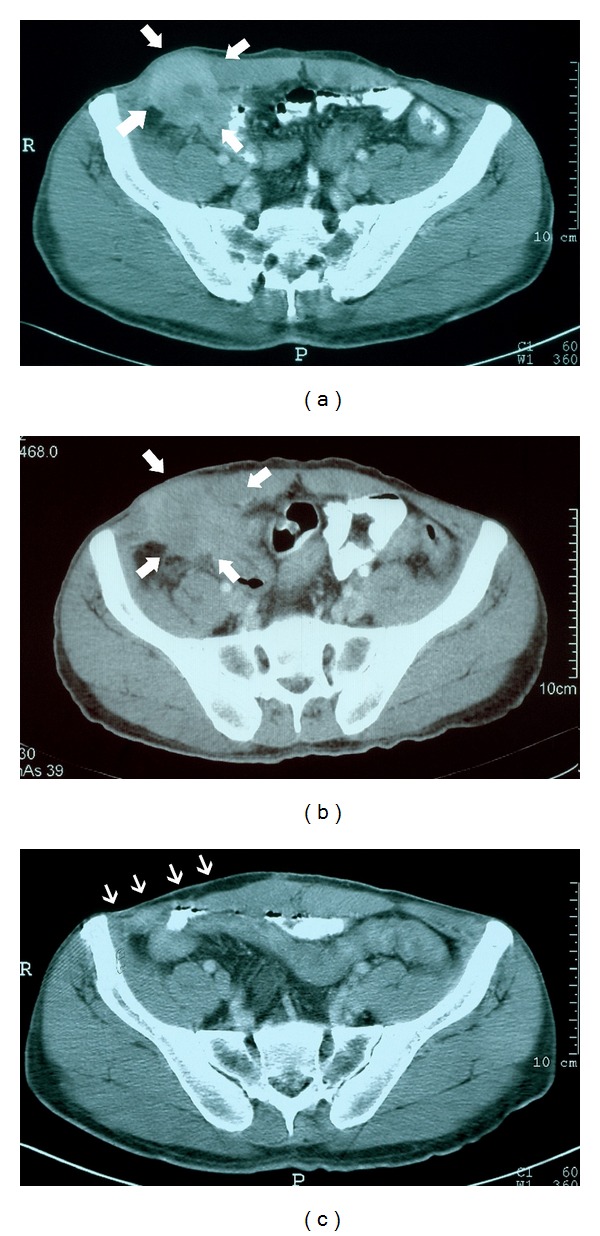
(a) The first CT of the patient before neoadjuvant chemotherapy. Thick arrows show the colonic mass, which invaded adjacent tissues including skin. (b) There was not enough mass regression after neoadjuvant therapy. (c) Excellent mesh adaptation at 6 months. Thin arrows highlight the mesh area.
